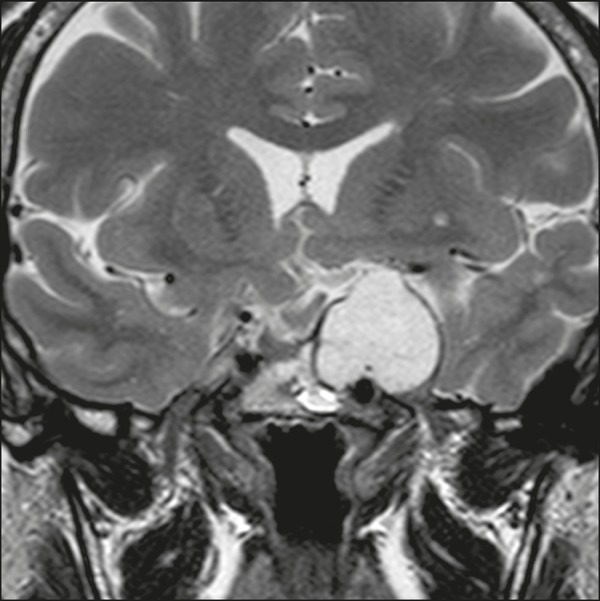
Hemangioma. Coronal T2-weighted MRI sequence showing a left juxtasellar
